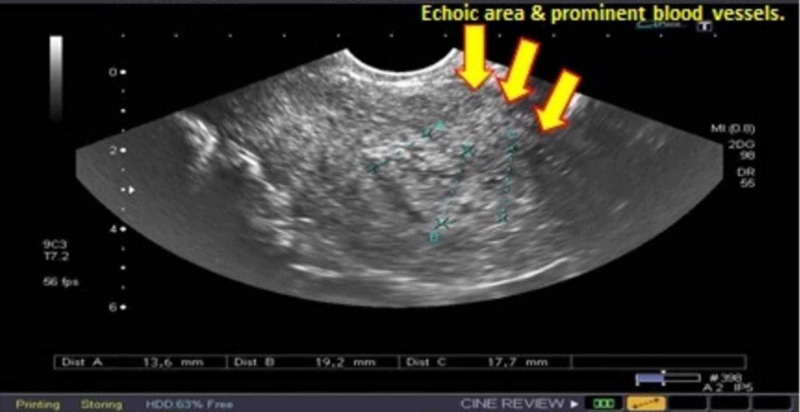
Pelvic ultrasound showing dilated blood vessels
